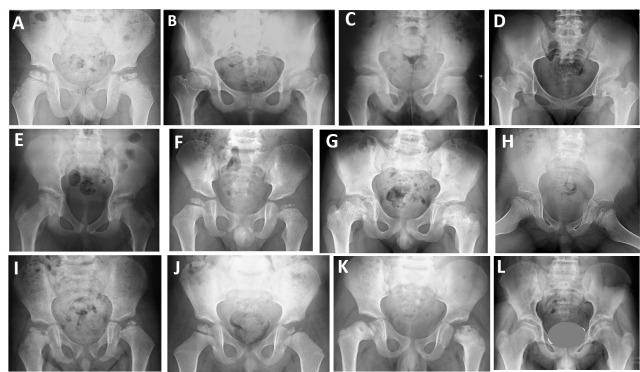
Radiographs of 12 patients without disease-causing variants. Patients with radiological features inconsistent with a specific skeletal dysplasia (A–G): severe irregularities of the proximal femoral epiphysis in P20 at 6 years of age (A); destruction of the femoral head in P21 at 16 years of age (B); flattening and irregularity of the bilateral femoral epiphyses in P22 at 9 years of age (C); a deformed femoral head and short femoral neck in P26 at 5 years of age (D); an irregular acetabulum and proximal femoral epiphyses in P33 at 11 years of age (E); a very small, irregular femoral head in P34 at 6 years of age (F); and irregular proximal femoral epiphyses in P38 at 8 years of age (G). Patients whose radiological features are consistent with LCPD (H–L): P23 at 3.5 years of age (H), P24 at 3 years of age (I), P32 at 4 years of age (J), and P36 at 4 years of age (K). Note the bilateral fragmented, small, irregular, or destroyed proximal femoral epiphyses. P28 at 15 years of age, with an ANFH phenotype, had irregular, flat proximal femoral epiphyses (L).
